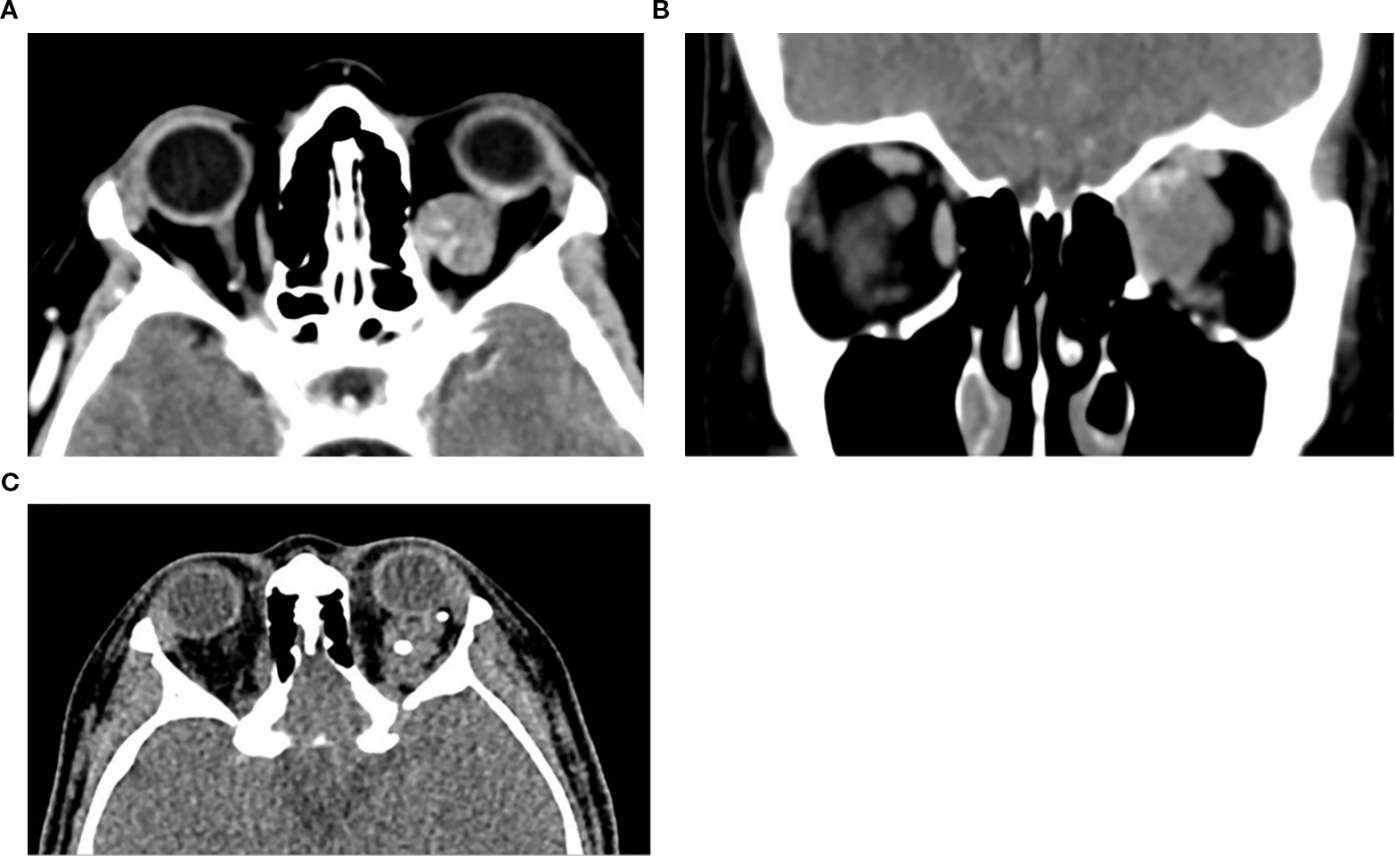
Axial (a) and coronal (b) post-contrast CT image of the orbits in a 65-year-old female patient demonstrates a well-defined, circumscribed, intraconal lesion in the left orbit located between the medial rectus muscle and the optic nerve, with associated proptosis and mild globe indentation. The lesion exhibits early focal stippled enhancement following contrast administration. These imaging features are characteristic of a CVM. (c) Axial non-contrast CT of a 16-year-old female patient with left orbital venous malformation, showing multiple well-circumscribed hyperdense round foci within the intraconal lesion, consistent with phleboliths, a typical finding in venous malformations.
